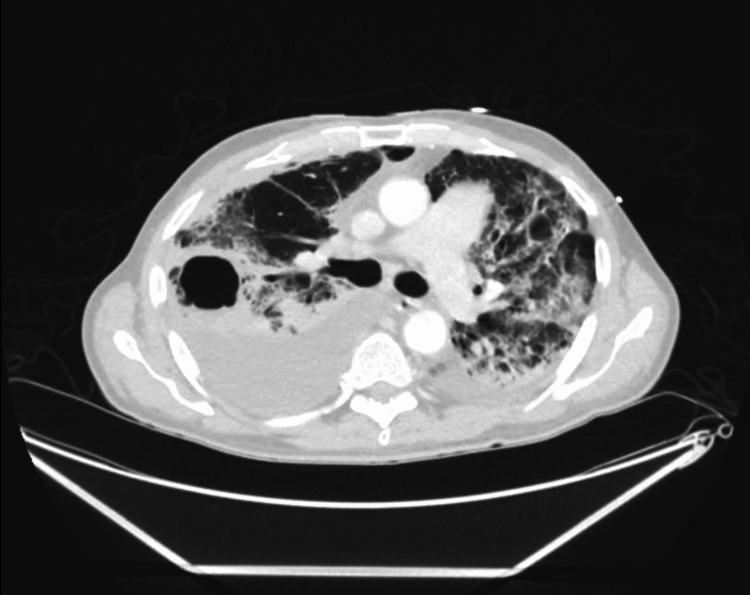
CT thorax on day 0 of current admission
